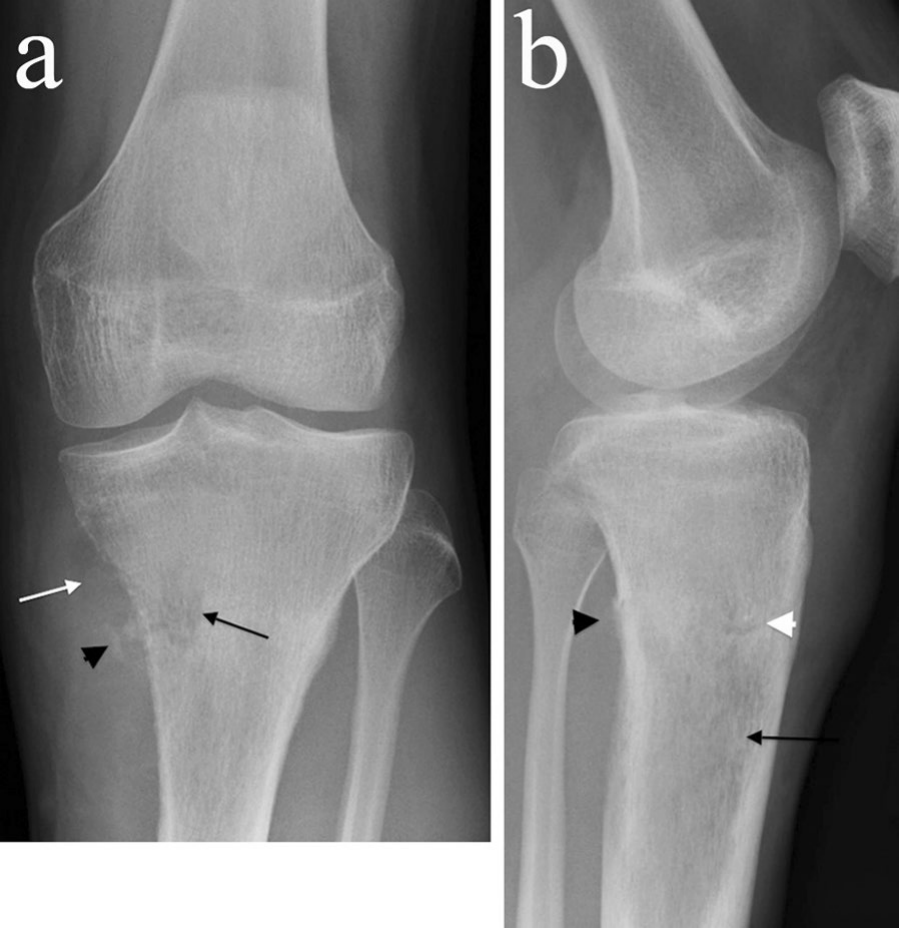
a AP and lateral b plain radiographs demonstrate an ill-defined radiolucent lesion in the proximal tibial metadiaphysis (black arrows) with posteromedial cortical destruction and adjacent ossification (black arrowheads). A medial soft tissue mass with low density raises the possibility of a fatty matrix (white arrow). A pathological fracture is present (white arrowhead)
